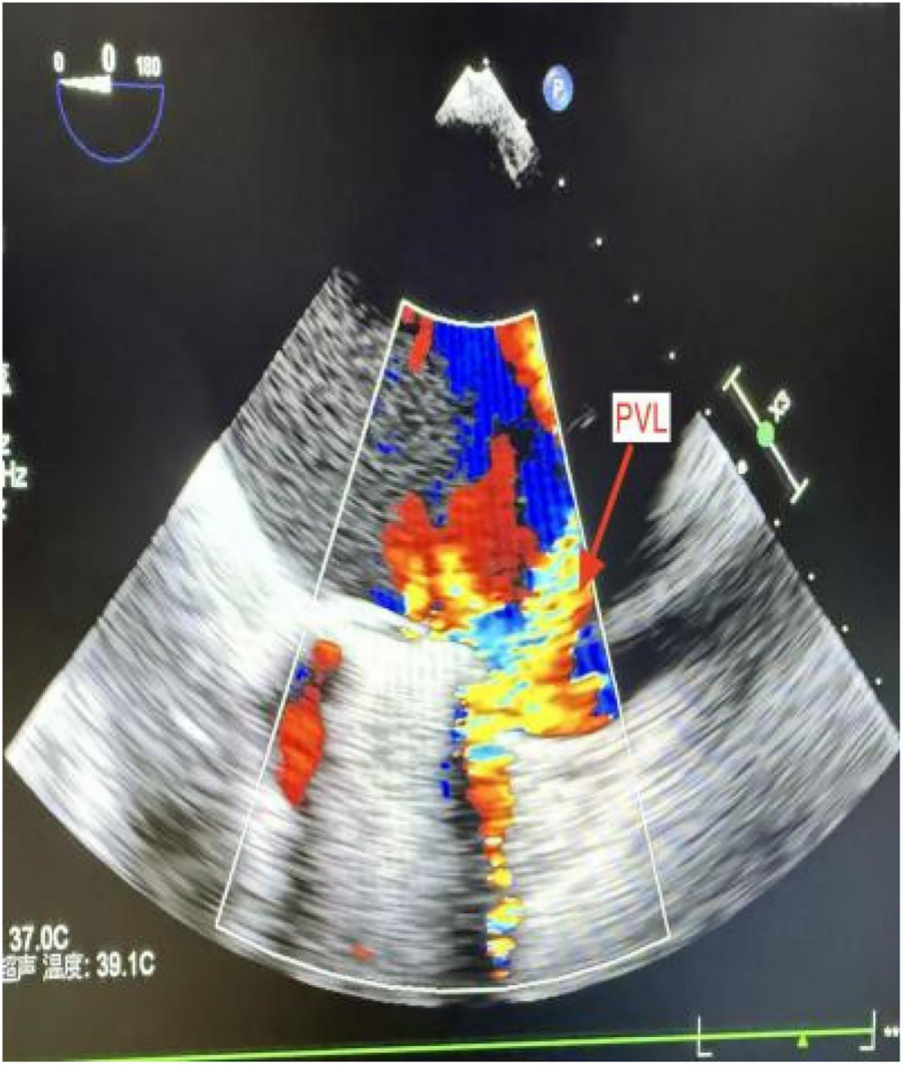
Transesophageal echocardiography (TEE) demonstrated a 5 mm PVL adjacent to the posterior mitral annulus.
